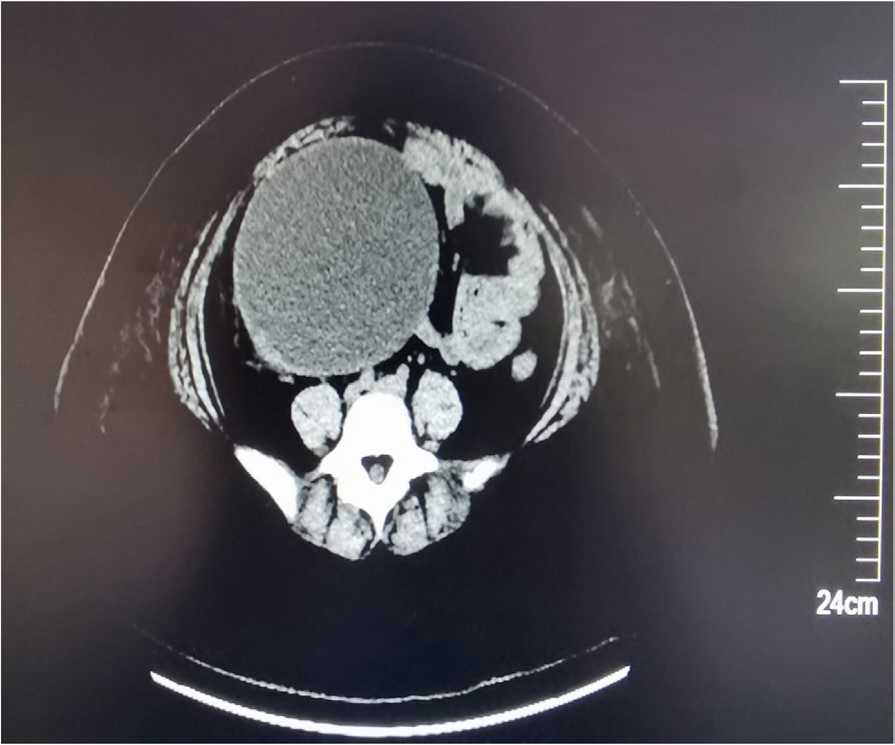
CT-scan: A huge ovarian cyst that occupies the entire abdominal and pelvic cavity, pushing and squeezing the intestinal tube in the abdominal cavity
